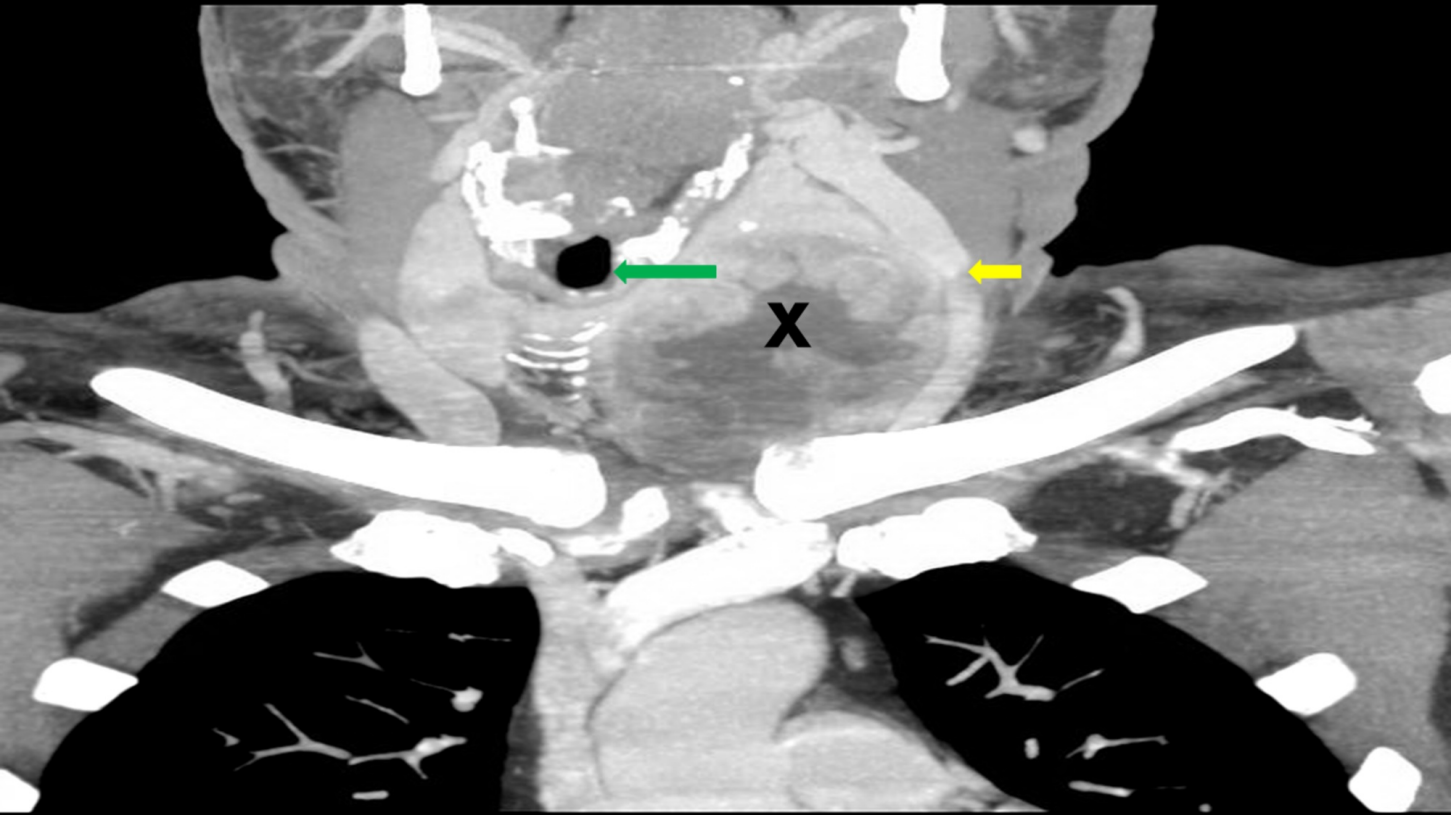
Computed tomography scan of the neck showing a necrotic thyroid mass (labeled X) on the left side, extending into the anterior mediastinum, and compressing the surrounding structures including the internal jugular vein (yellow arrow). Also visible is the trachea (green arrow) which is deviated towards the right side.
